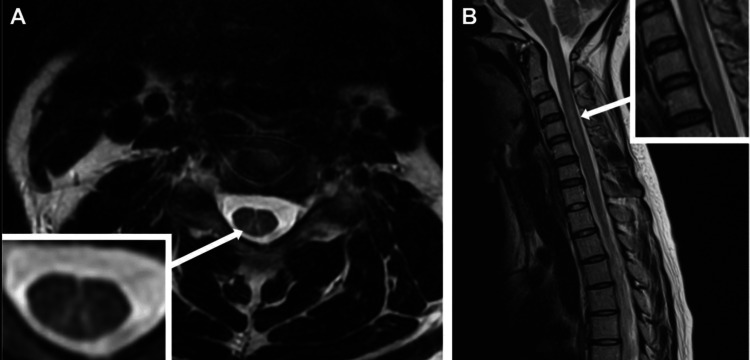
Typical MRI features of subacute combined degeneration of the spinal cord associated with nitrous oxide misuse. Axial T2-weighted image (A) reveals a hyperintense signal within the dorsal columns of the cervical spine, forming the characteristic “inverted V” pattern (arrow). Sagittal T2-weighted image (B) demonstrates longitudinally extensive dorsal column hyperintensity, spanning multiple cervical segments (arrow). Image credit: Paris et al. (2023) [8], under CC BY-NC 4.0 license. MRI, Magnetic resonance imaging
